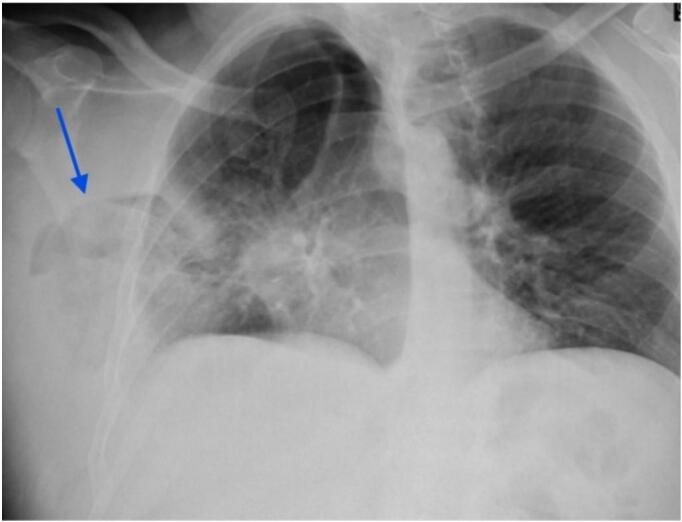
The Chest X-ray (PA view) showed a triangular lucency (blue arrow) lateral to the right 4th and 5th ribs, indicating lung protrusion beyond the thoracic cage. (For interpretation of the references to colour in this figure legend, the reader is referred to the web version of this article.)
